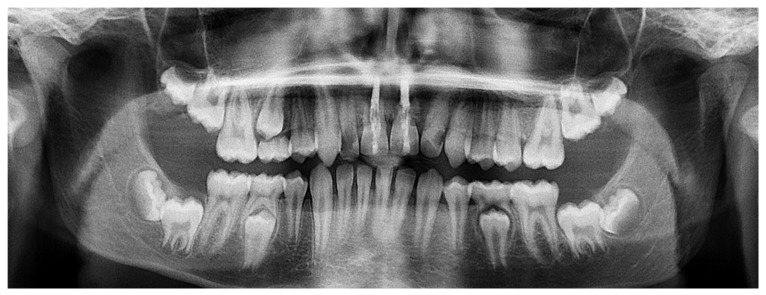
Orthopantomogram of the patient’s dental condition in April 2018.
